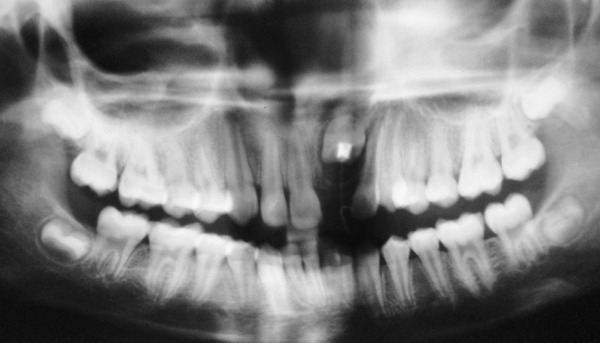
One week postoperative panoramic radiograph revealing proper placement of bracket and absence of any radiopaque masses
